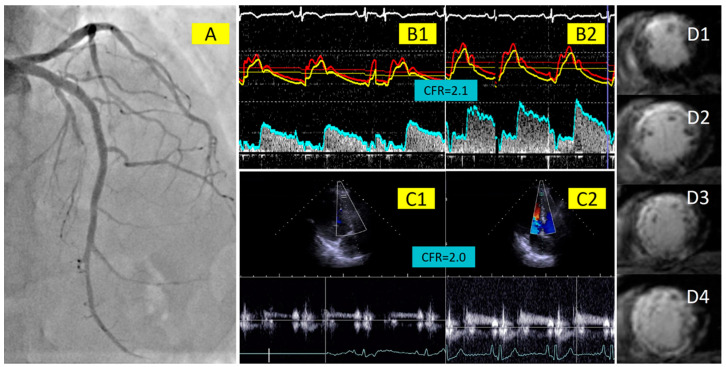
Representative images showing the recanalized LAD artery (A) with invasive Doppler wire-based assessment of coronary flow velocity at rest (B1) and in hyperemia (B2), and the corresponding echocardiography-derived measurements of coronary flow velocity at rest (C1) and during hyperemia (C2), which correlate with the degree of myocardial fibrosis from the mid anterior wall to the apex of the left ventricle on cardiac magnetic resonance imaging (D1–D4).
